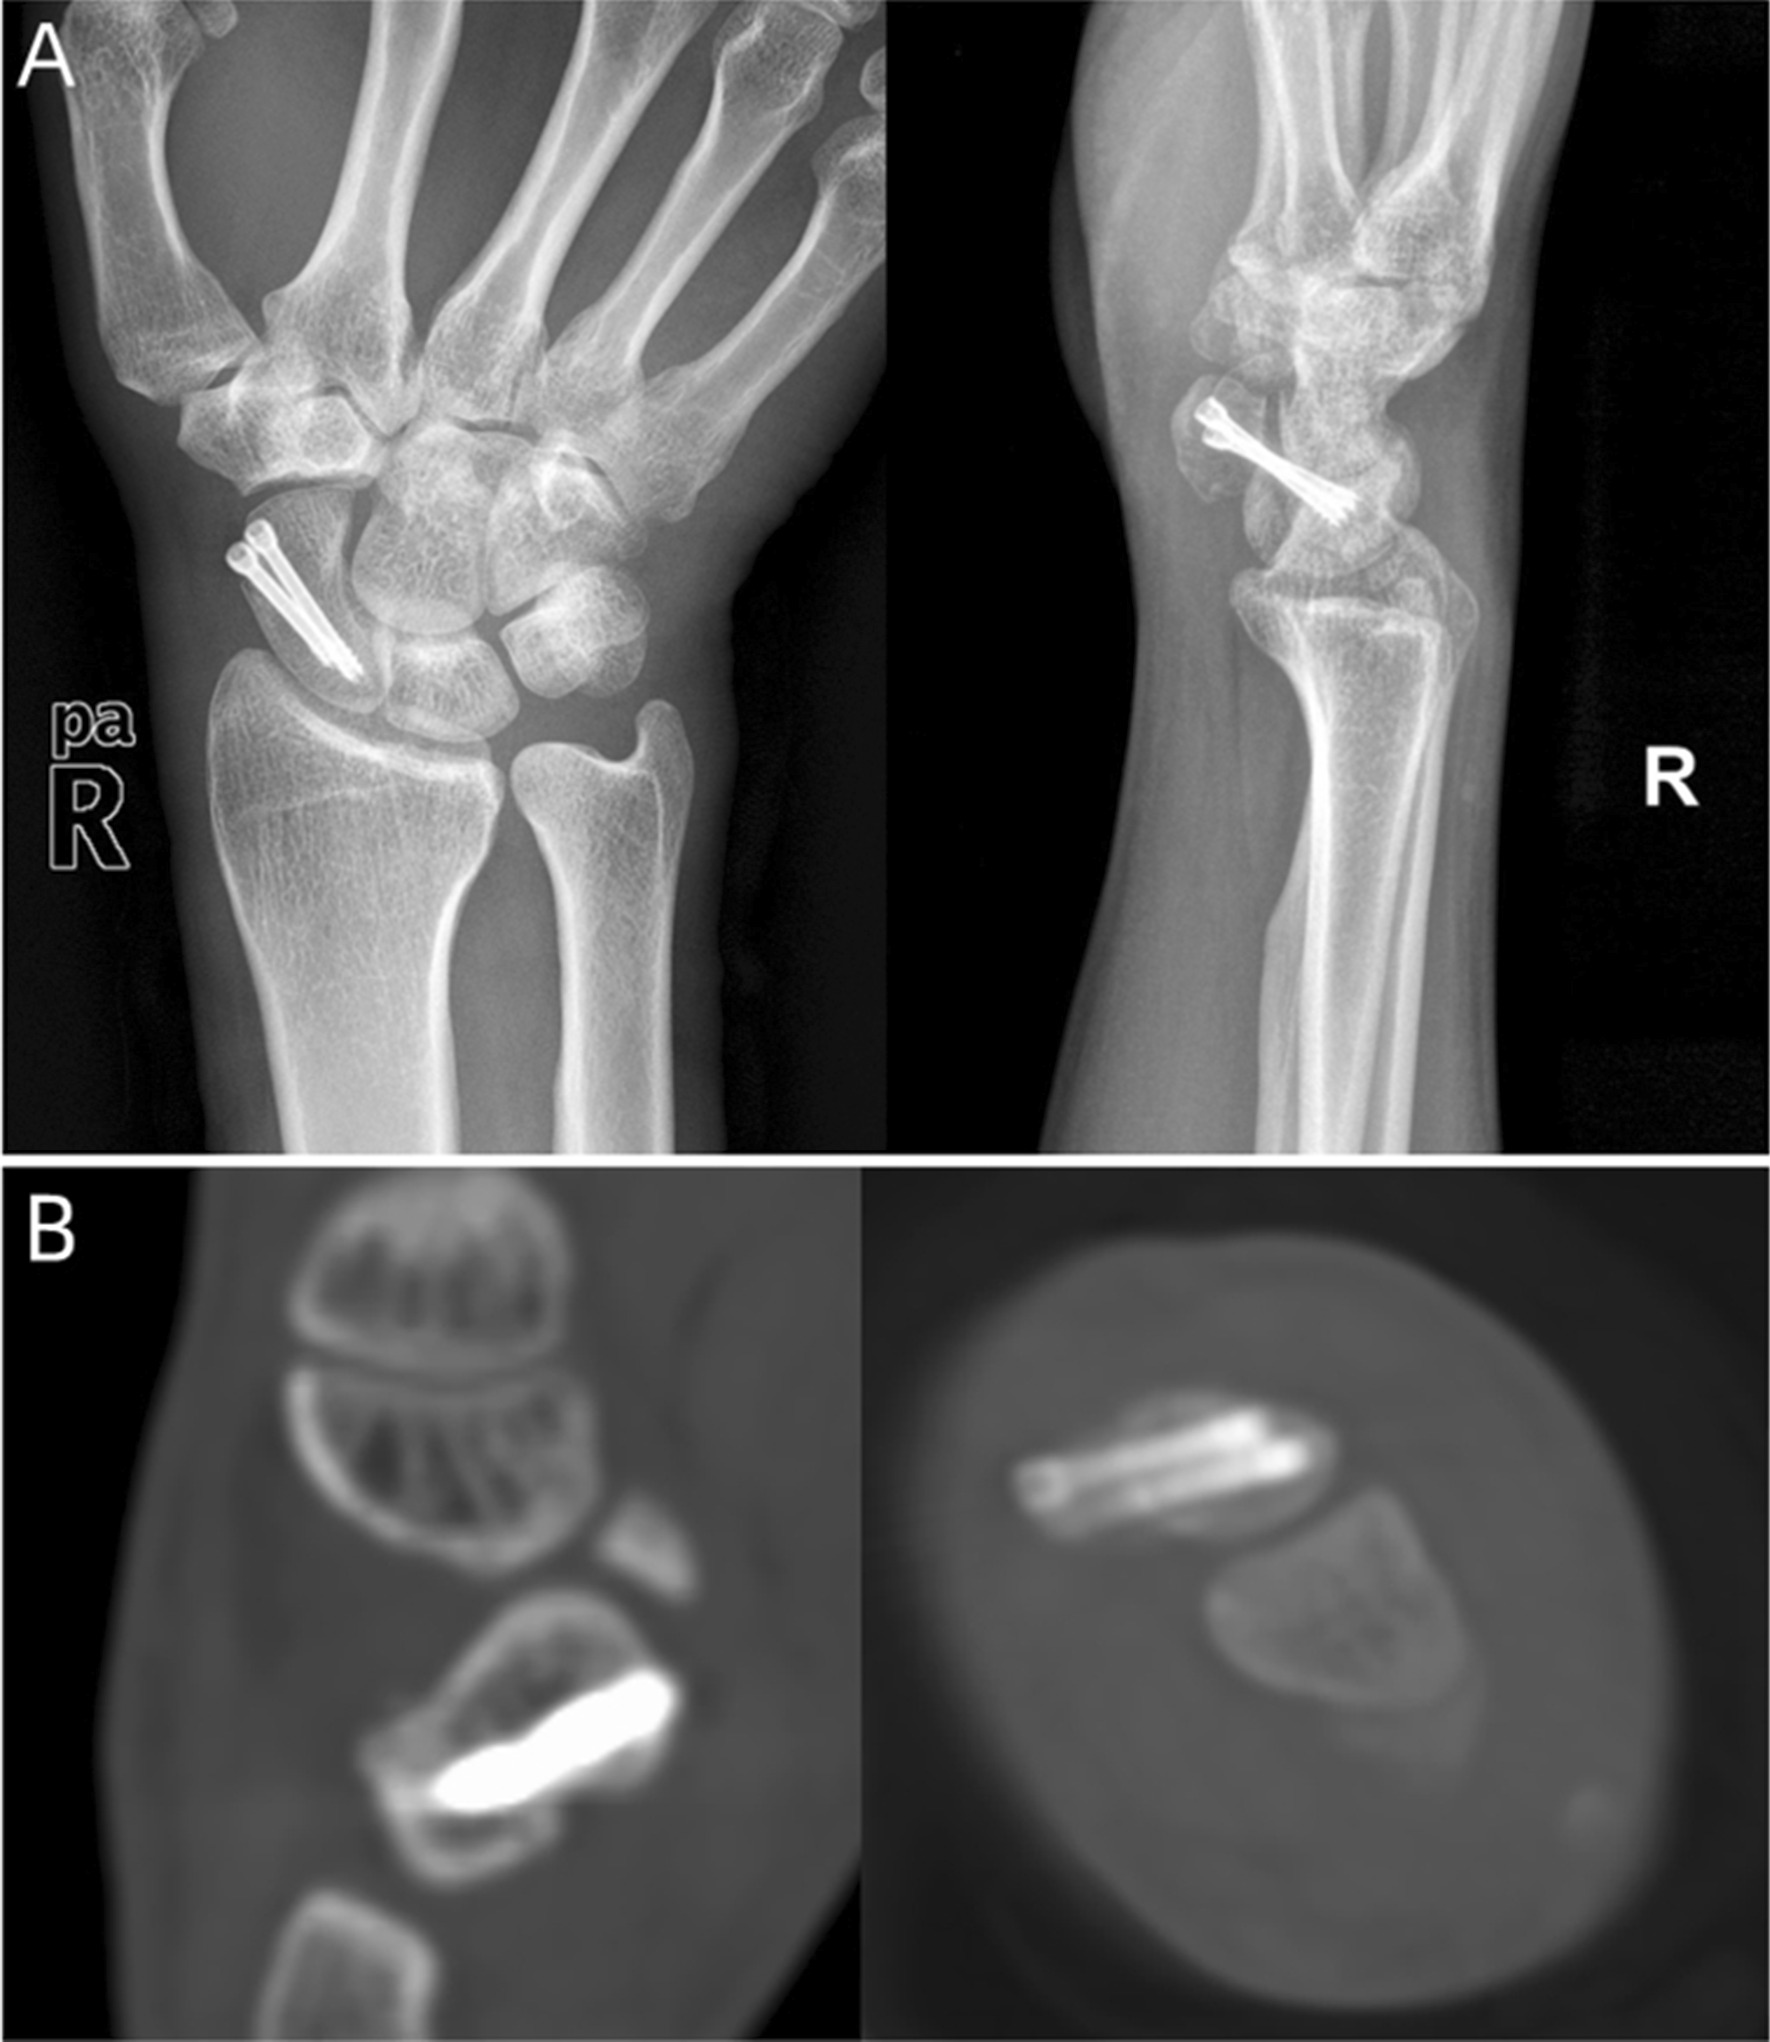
Union was achieved at 12 weeks after surgery, which was assessed by plain radiograph (A) and CT scan (B)
